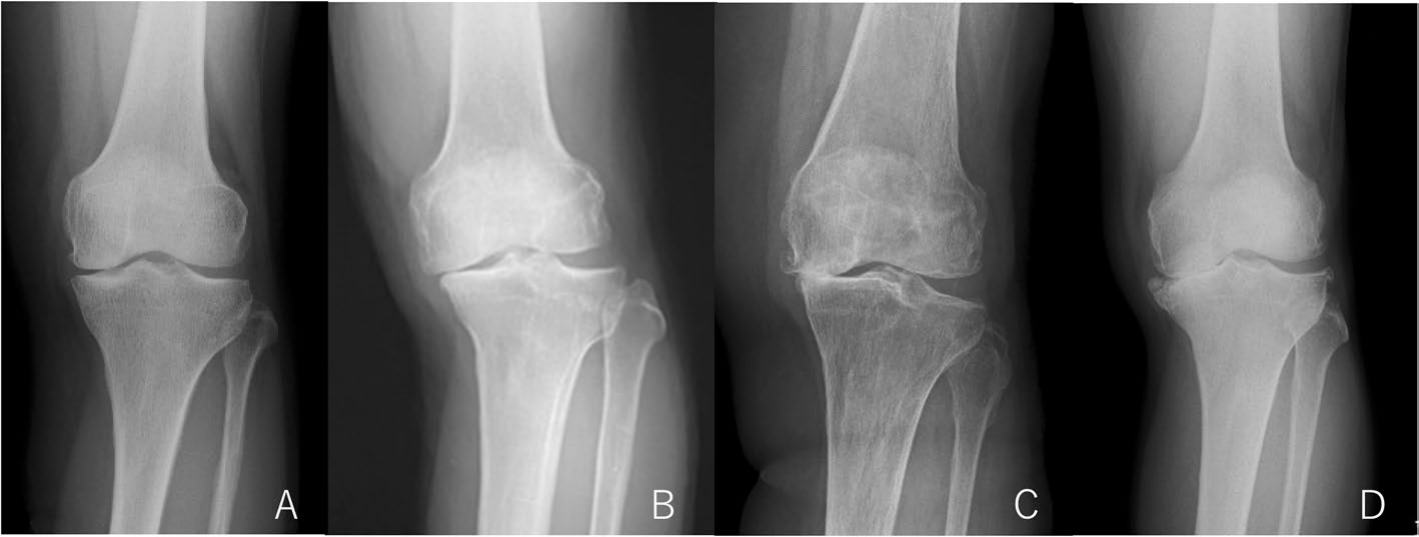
Ahlbäck radiographic classification grade
